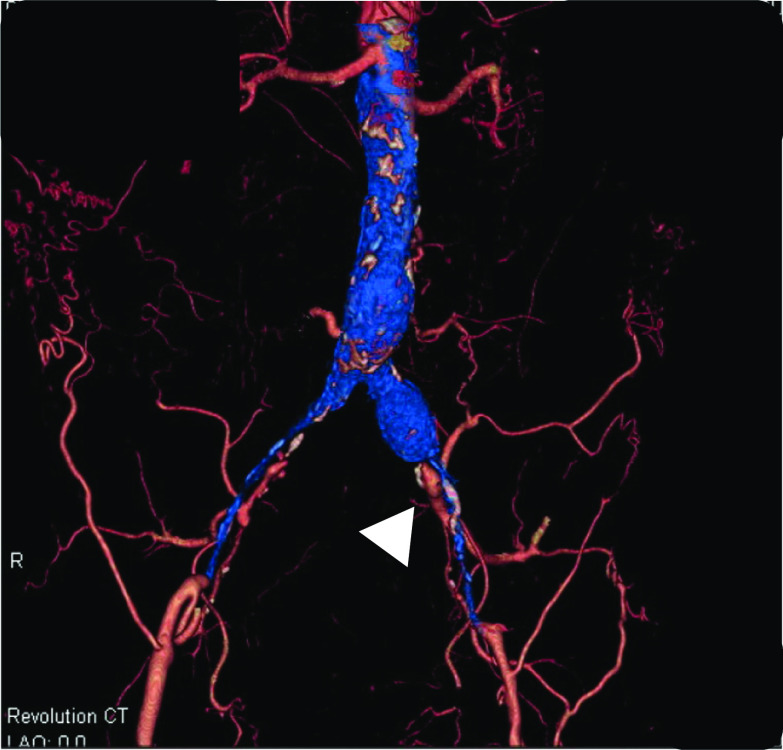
CT angiography revealed the left internal iliac artery was also not completely occluded (white arrowhead)
